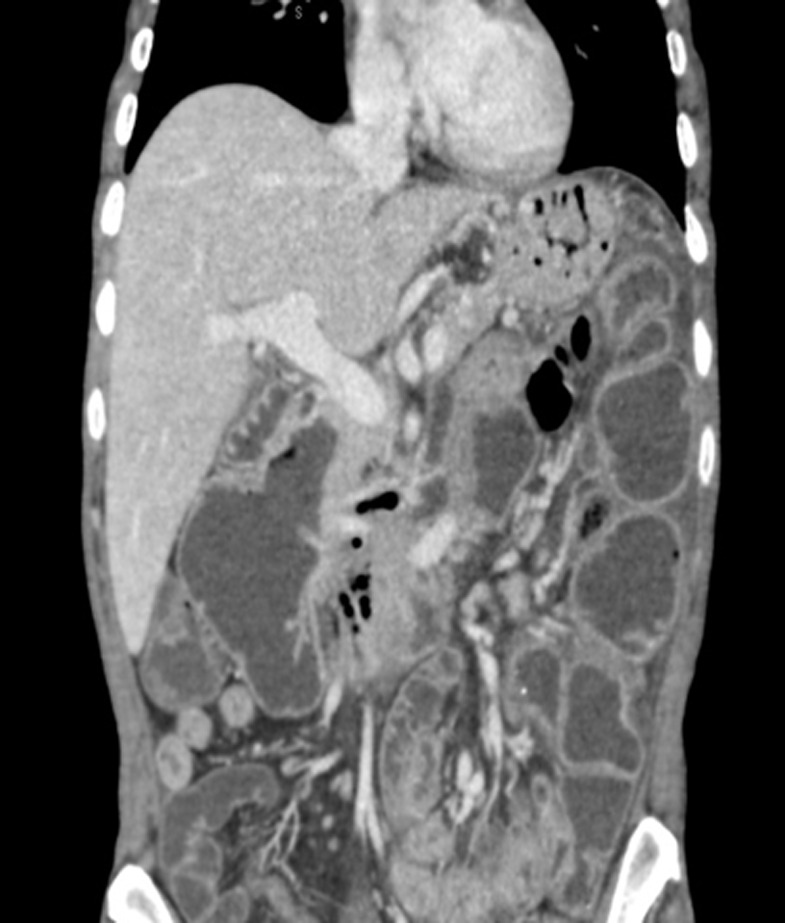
computed tomography showing distended proximal small bowel loops with multiple diverticula
